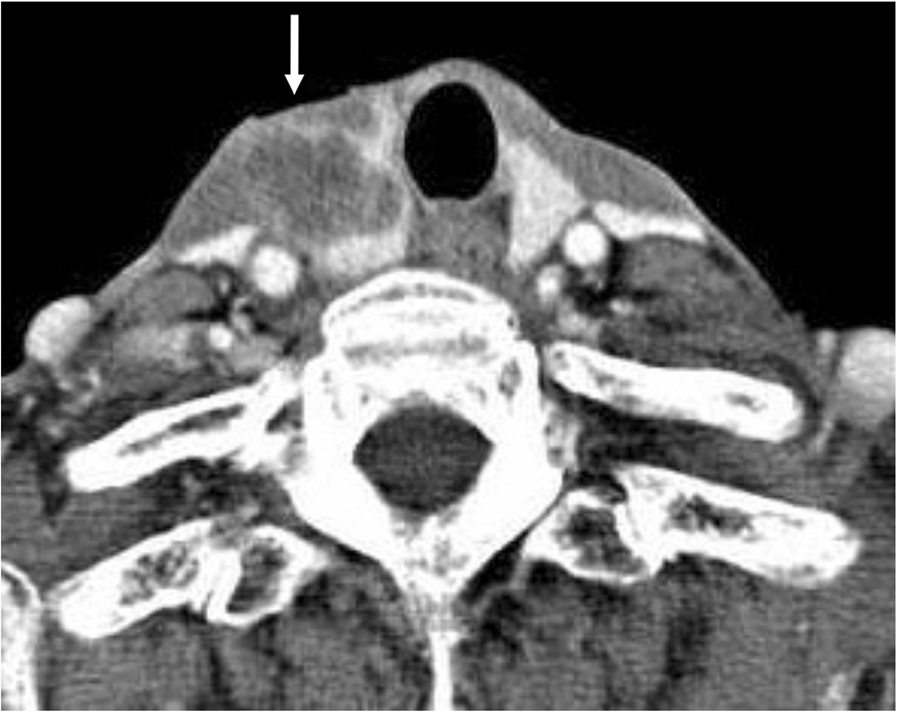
Computed tomography of mass in the thyroid gland. The invasion of muscles in the anterior region is indicated with an arrow
